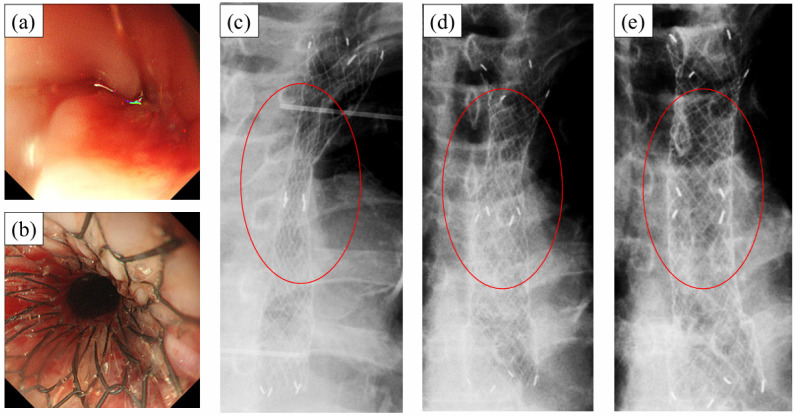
Esophageal stenting to the case after definitive chemoradiotherapy (dCRT). (a) esophageal stenosis due to recurrence of tumor after dCRT (b) Endoscopic findings immediately after self-expanding metal stents (SEMS) placement. Although the esophageal lumen became visible, the stent showed only minimal expansion. (c) Chest radiograph obtained on the same day after SEMS placement (red circle) showing persistent severe luminal narrowing due to incomplete stent expansion. (d) 1 day after stenting, substantial stent expansion, although residual waist formation remained at the stenotic segment. (e) 7 days after stenting, complete resolution of the stenosis with full stent expansion.
